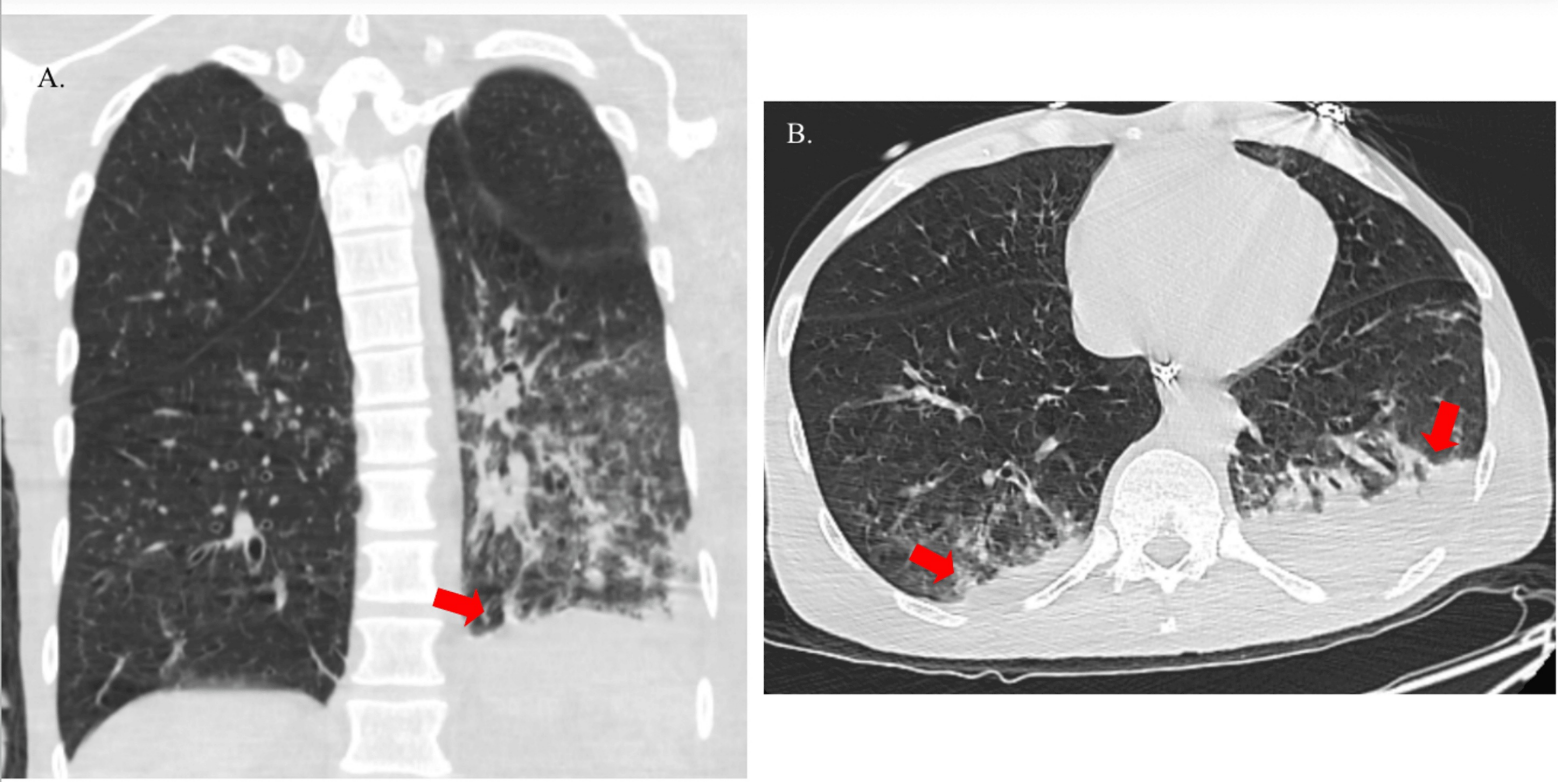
CT chest without contrast showing bibasilar consolidations (arrows). The images show (A) coronal view and (B) axial view.
